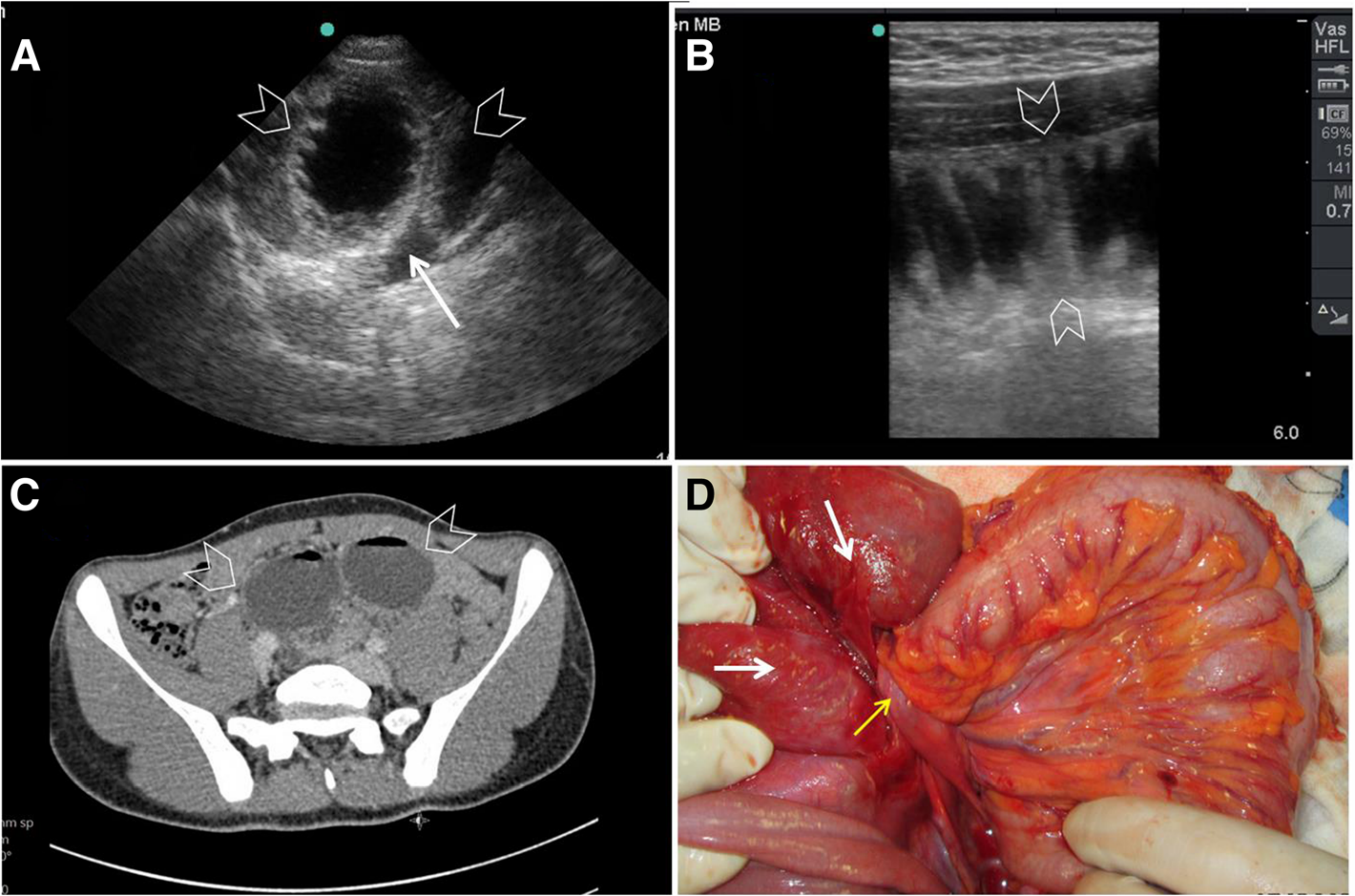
A 12-year-old boy presented with abdominal pain and vomiting. On examination, the lower abdomen was not distended, soft, but tender. Bowel sounds were reduced. POCUS examination revealed two non-functioning jejunal loops (arrowheads) (a–b) as evidenced by the valvulae conniventes of the loops. Abdominal CT scan confirmed the same findings (c). The patient did not respond to conservative management and needed surgery. Laparotomy (d) has shown the two jejunal loops (white arrows) obstructed by a congenital fibrous band (yellow arrow)
